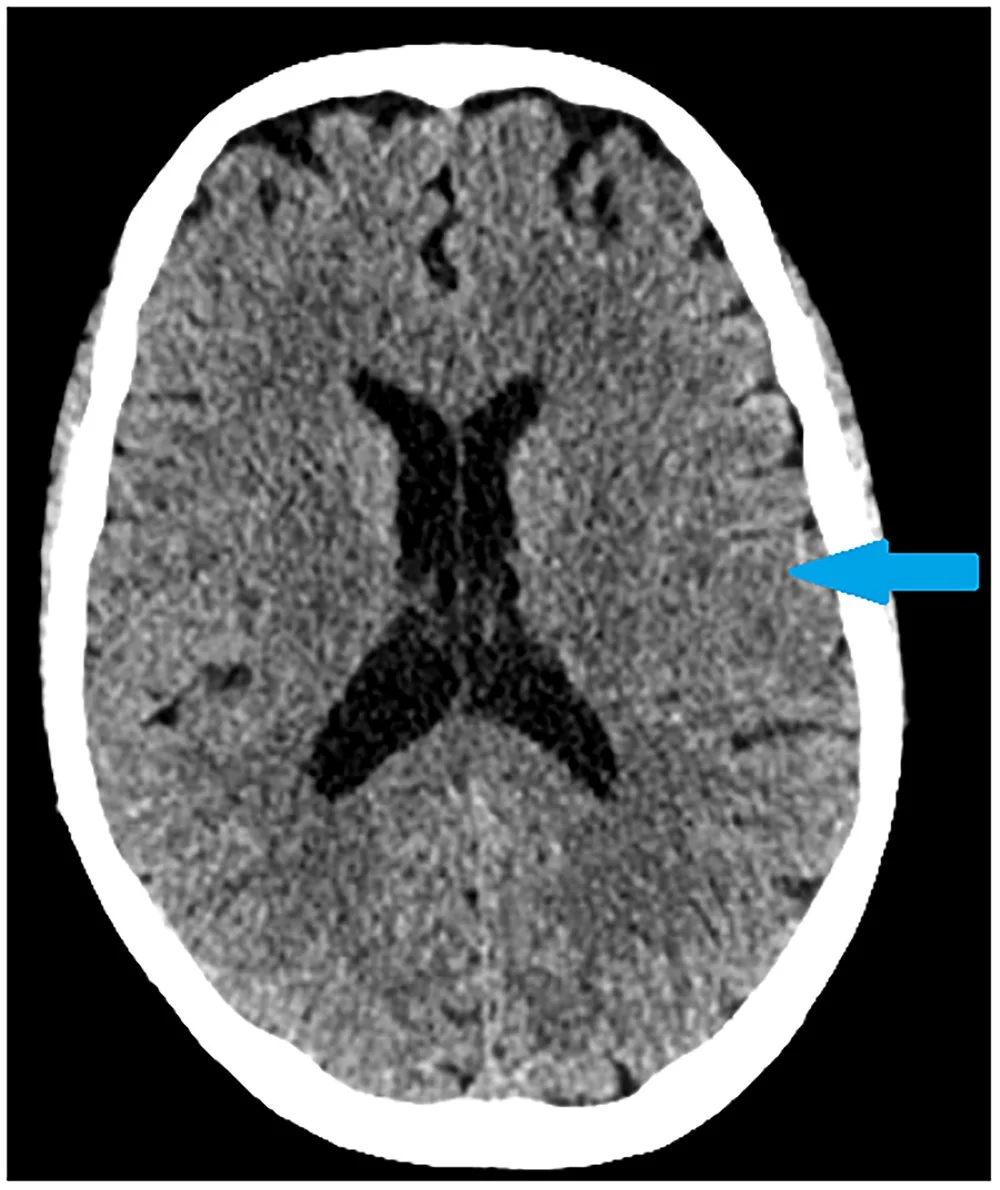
Non-contrast axial brain CT demonstrating a cortico-subcortical hypodensity within the left middle cerebral artery territory, consistent with an acute ischemic infarction.
